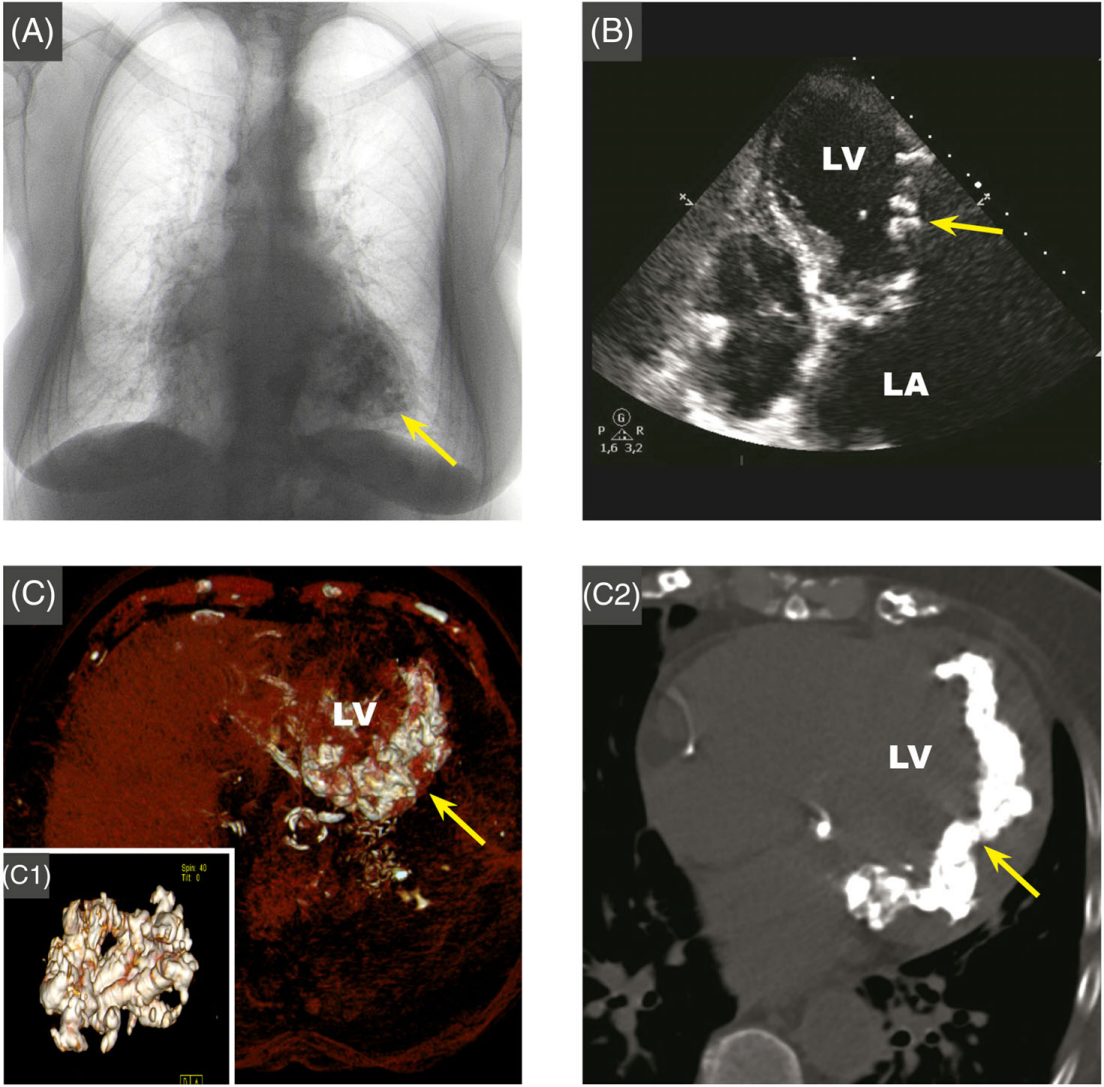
(A) Chest X‐ray showing a diffuse hypodense lobulated area in the left ventricle. (B) Video 1. 2D‐Echocardiogram revealing diffuse aortic and mitral calcifications with mild stenosis, marked septum and antero‐lateral asymmetric hypertrophy, with extensive antero‐lateral calcifications. (C) C1: CT‐VRT reconstruction of the left ventricle, respectively axial view and focus on the coronal view. C2: Video 2. Non‐contrast CT MPR‐axial view presents widespread calcifications in the left ventricular wall extended to the mitral‐aortic annulus and both coronary arteries
